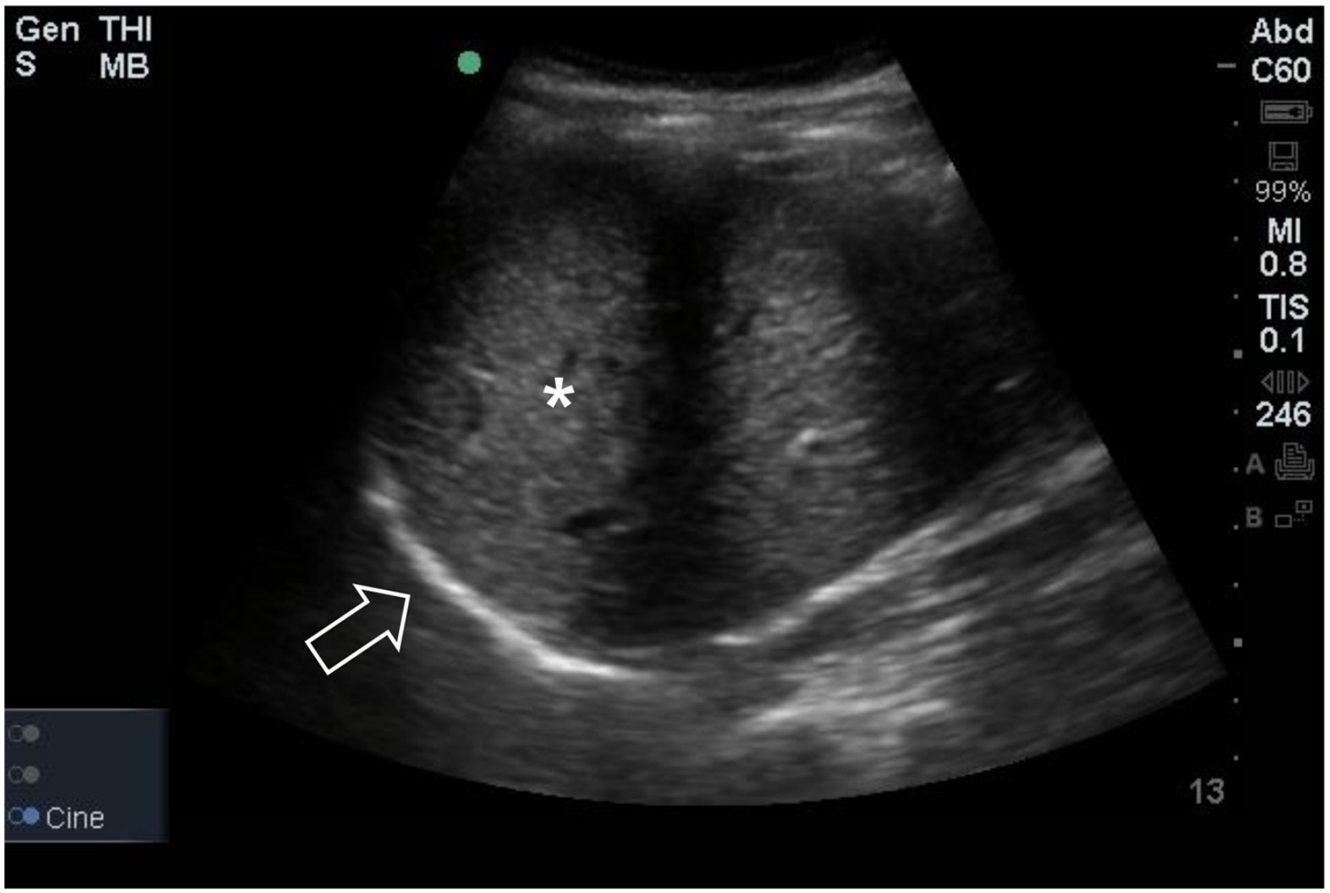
Diaphragmatic excursion as measured using 2D mode sonography. With the probe fixed on the right midaxillary line at approximately the sixth to ninth intercostal spaces, the ultrasound beam was directed to the hemidiaphragmatic domes at an angle of about 70 degrees. The diaphragm (white arrow) is located cephalad to the liver <asterisk>.
